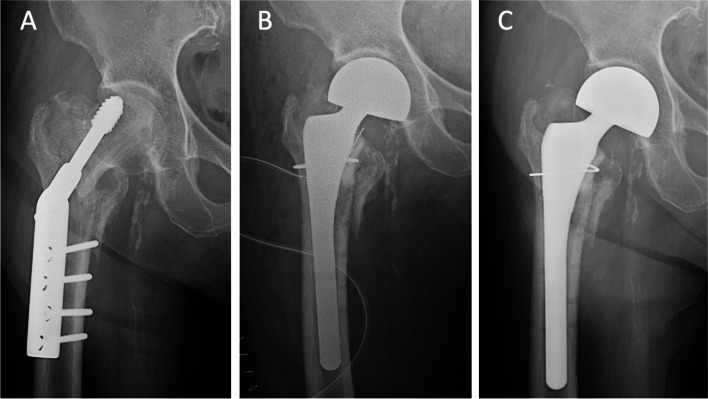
(A) 74-year-old female, with early fixation failure (postoperative 6 weeks, fracture collapse) of fixation for intertrochanteric fracture, (B) bipolar hemiarthroplasty with cementless primary stem, immediate postoperative radiograph, (C) postoperative 36 months
